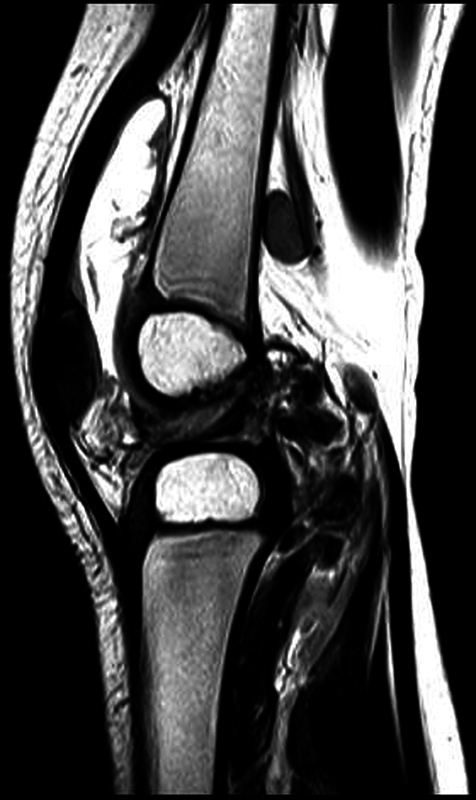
Sagittal, T1-weighted magnetic resonance image of the right knee (February 17, 2020) showing joint effusion, synovitis, enlarged lymph nodes, and oval formations in the posterior region.
